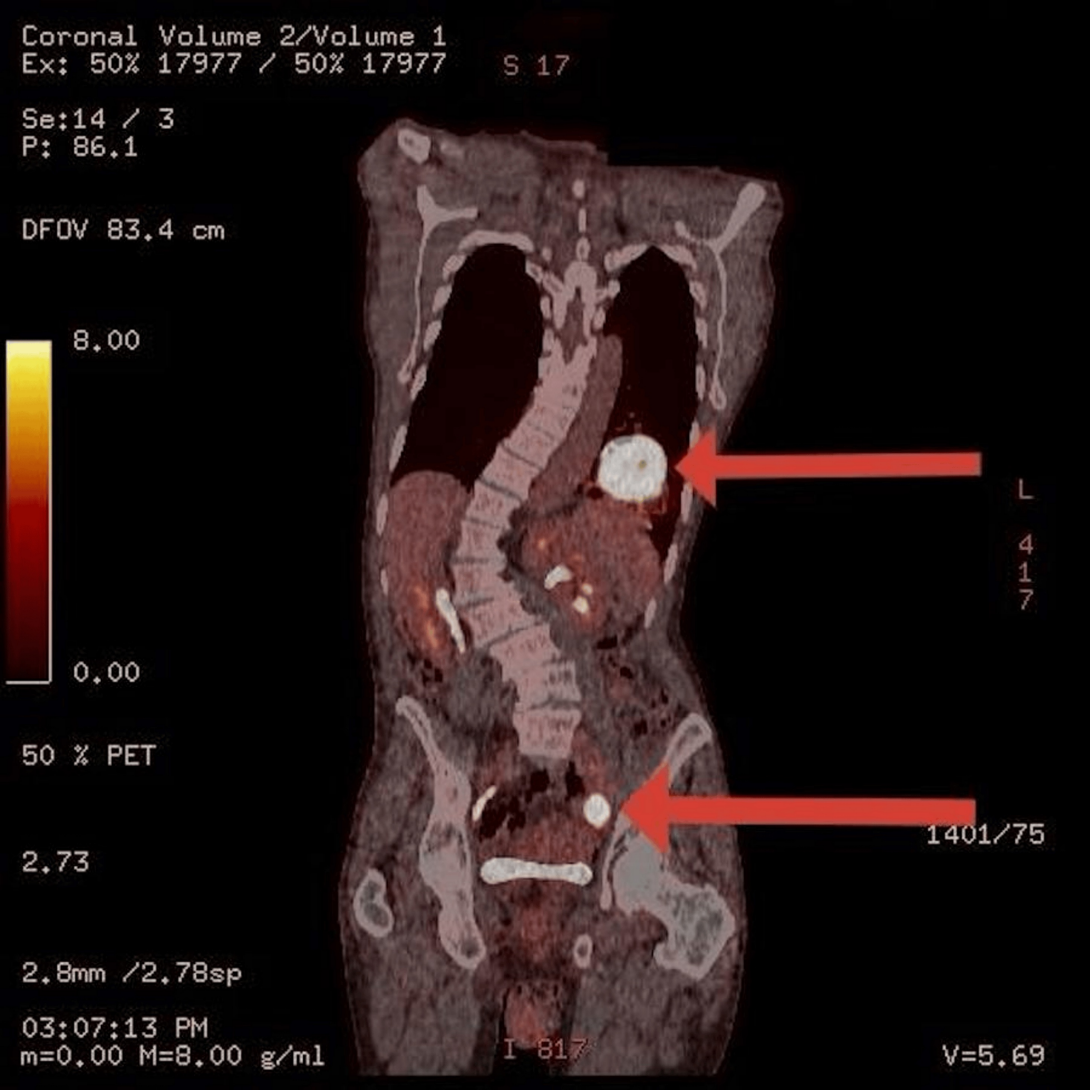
A PET-CT scan image demonstrating a metabolically active necrotic left lower lobe mass and left internal iliac lymph node, as indicated by the red arrows PET - positron emission tomography
